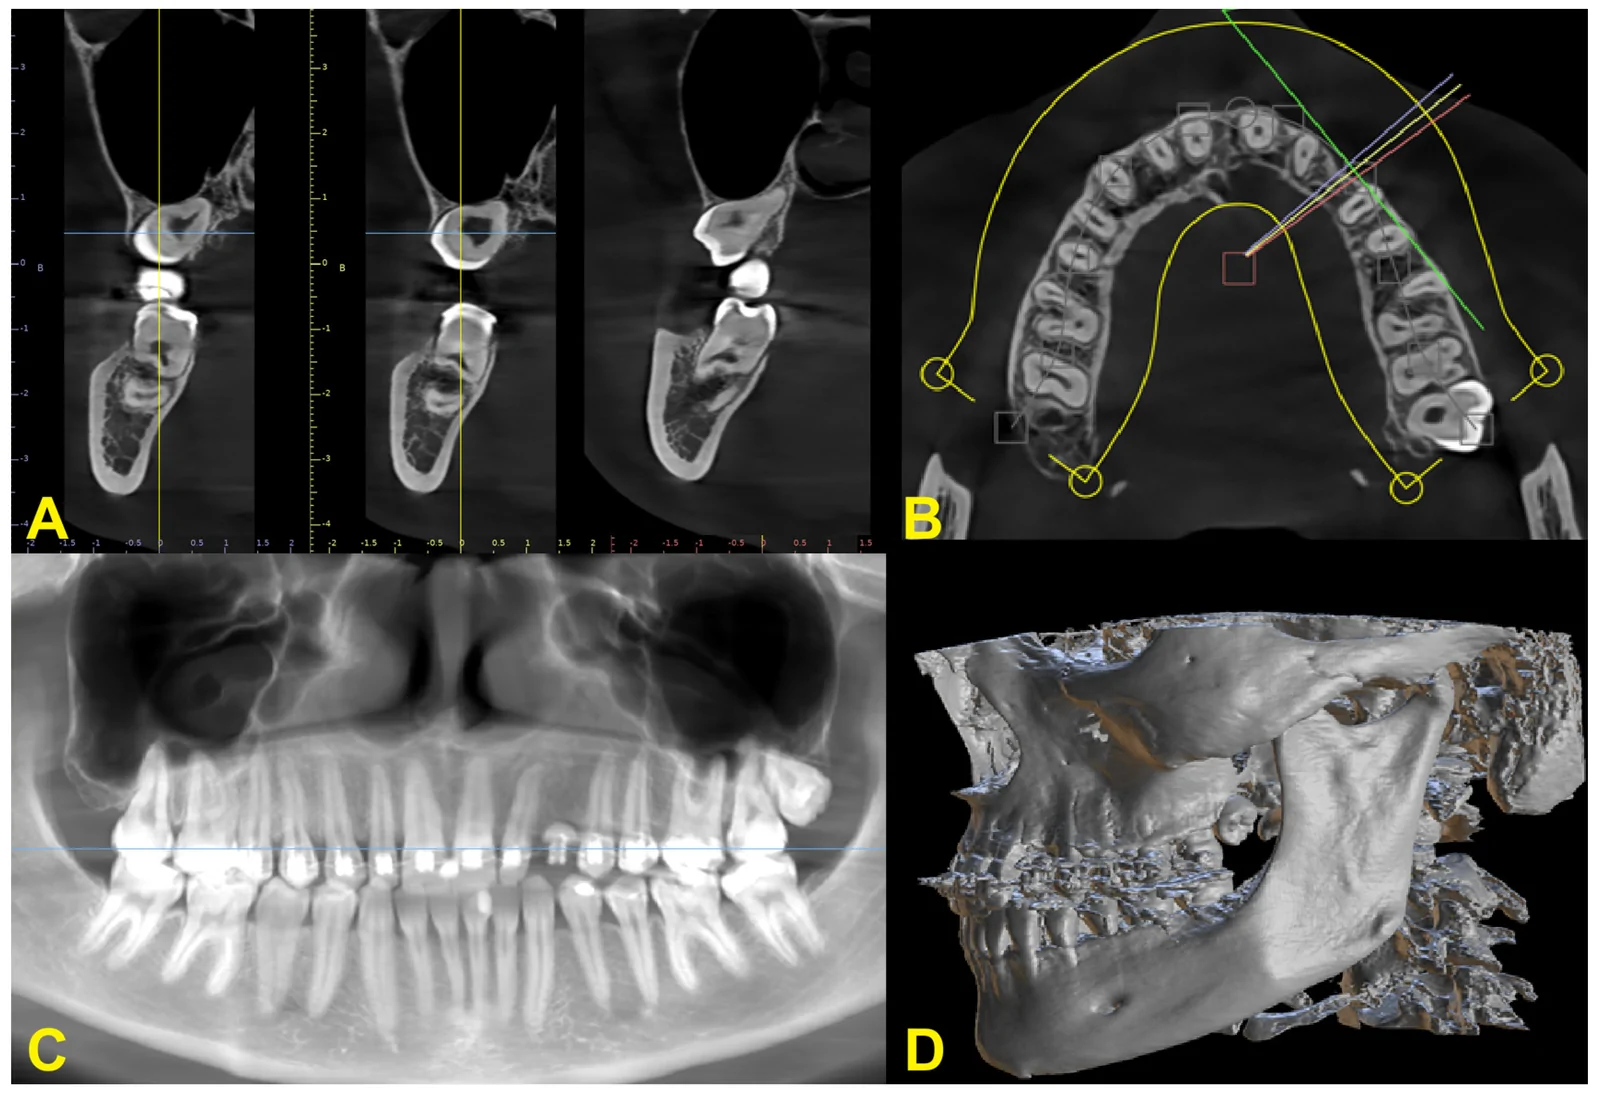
Cone-beam computed tomography of maxillary left third molar: (A) cross-sectional views; (B) axial view; (C) orthopantomographic view; (D) pseudo-3D reconstruction.
